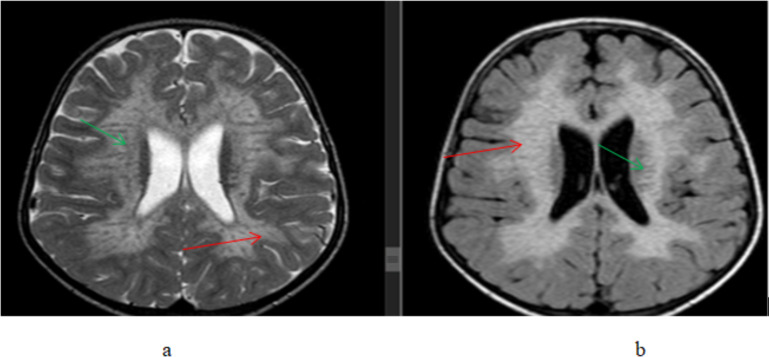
A 3-year-old female patient presented with developmental delay, (a) T2W axial and (b) FLAIR axial, brain MR images showing bilateral periventricular white matter hyperintense signal changes (red arrows) with sparing of subcortical U fibers and internal linear hypointensities(green arrows) giving “tigroid pattern”; The imaging features are those of metachromatic leukodystrophy
